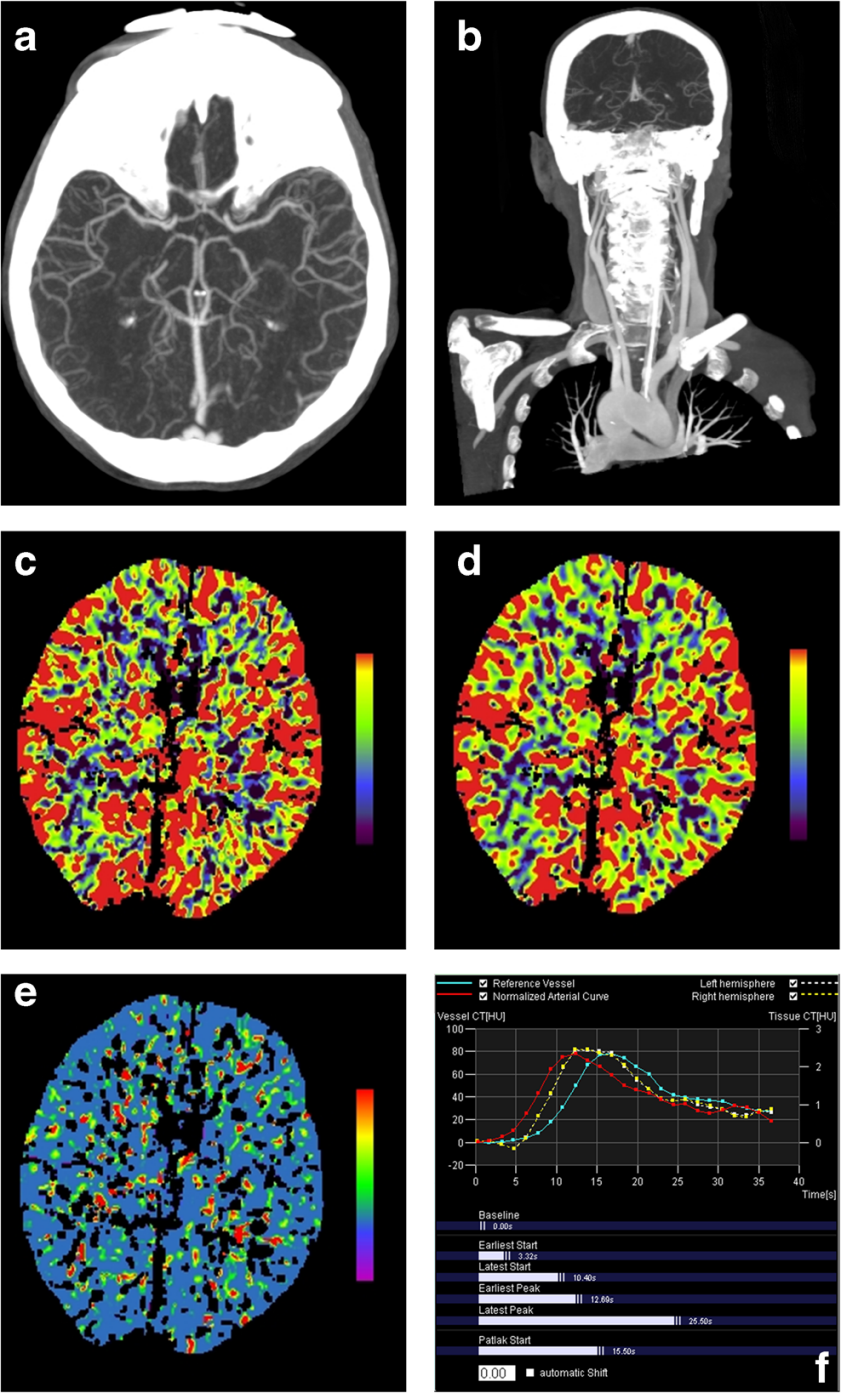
CTA and CTP via IO access. a Maximum intensity projection (MIP) axial reformatted image of the cerebral arteries. b Maximum intensity projection (MIP) coronal reformatted image of the supra-aortic branches. CTP post-processed maps of relative cerebral blood flow (CBF, c), cerebral blood volume (CBV, d), and mean transit time (MTT, e). (f) Technical adequacy confirmed by normal arterial input and venous outflow function curves with an adequate baseline and rapid upslope
